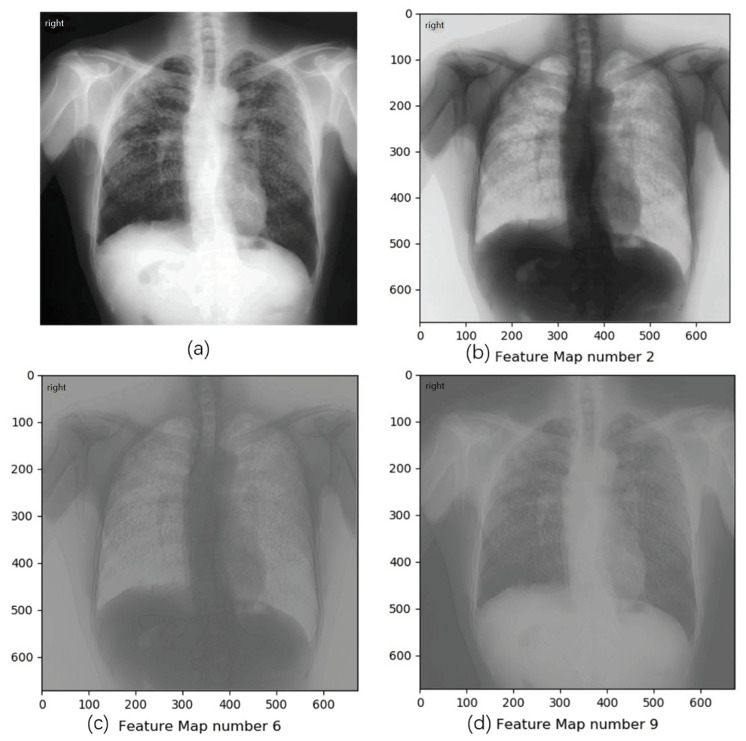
A pneumoconiosis-positive sample in the dataset and visualizations of feature maps from several channels in DenseNet53. (a) The original radiograph; (b) the 2nd feature map, which separates lung region to a certain extent; (c,d) 6th and 9th feature map, which capture and highlight suspected opacities in pneumoconiosis radiograph.
